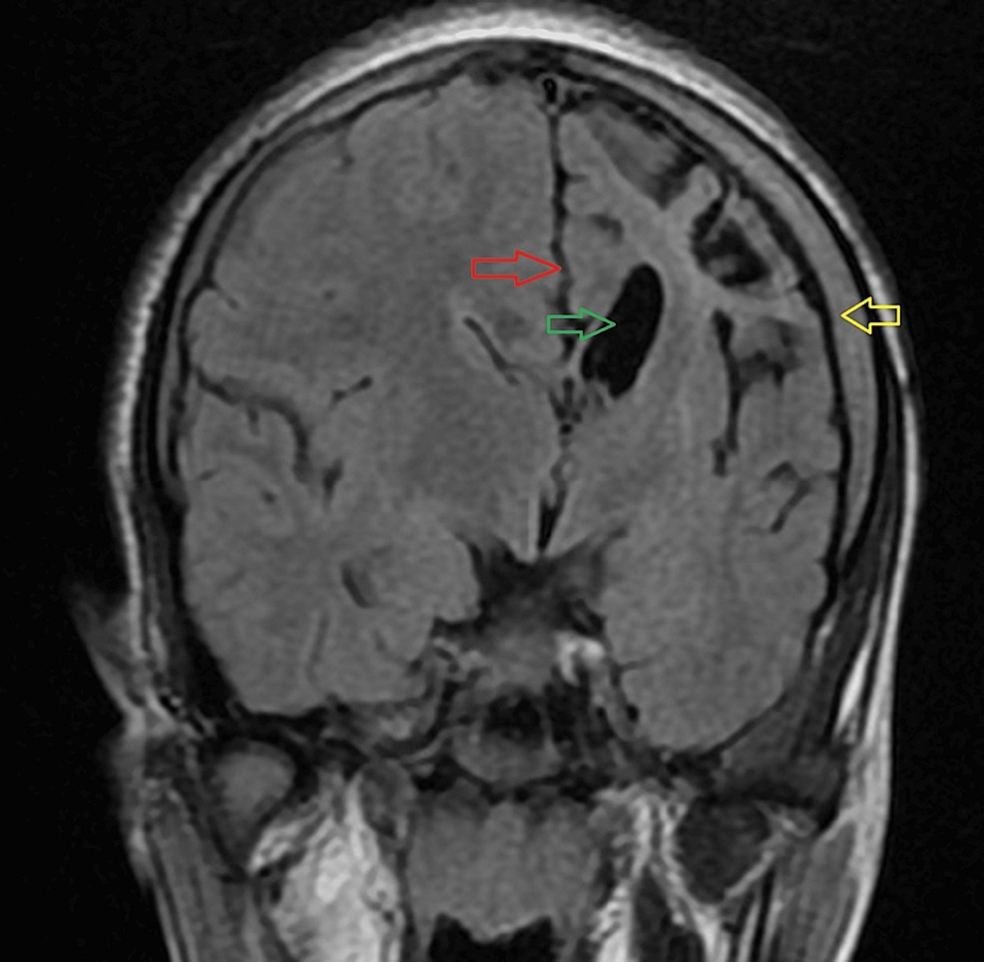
Coronal T2 FLAIR (fluid-attenuated inversion recovery) sequence of MRI of the brain showing calvarial thickening on the left side (yellow arrow) and ex-vacuo dilatation of the ipsilateral lateral ventricle (green arrow). Ipsilateral displacement of the falx is seen (red arrow). MRI: magnetic resonance imaging
